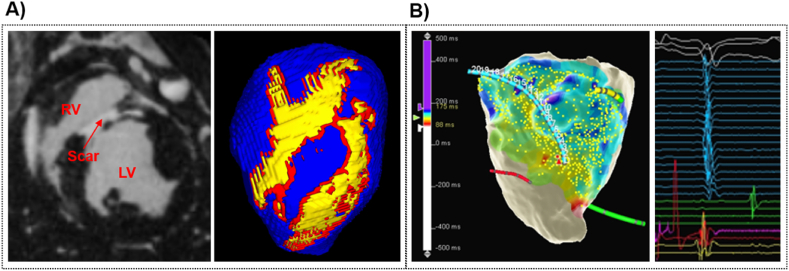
Experimental data. A) Example of LGE-MRI, showing the LV, RV, and the scar, with the corresponing LV (blue) segmentation, including scar (yellow) and border zone (red). B) Example of EP data acquired in-vivo using Precision™. Showing LAT map on the LV cavity computed by Precision™, and examples of acquired intra-cardiac EGMs (right).
